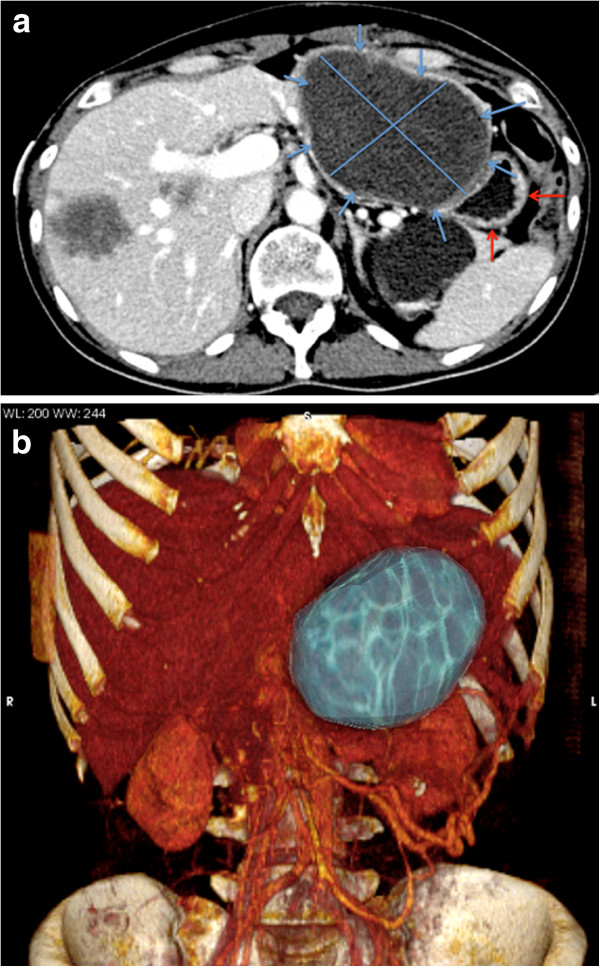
CT of patient before treatment. (a) Contrast-enhanced multi-detector CT. A 10 cm × 7 cm liver lesion (blue arrows with main diameters) completely occupying the left lobe in a multi-deposit disease is compressing and dislocating the stomach (red arrows). (b) 3D rendering image pre-treatment.
